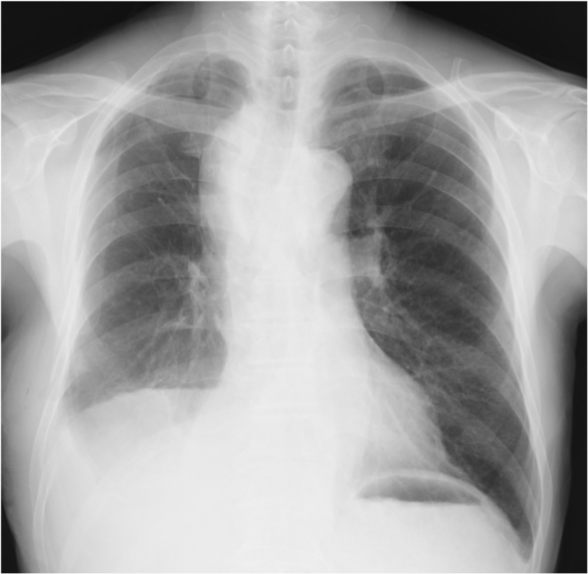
Chest radiography showing upper mediastinal mass
